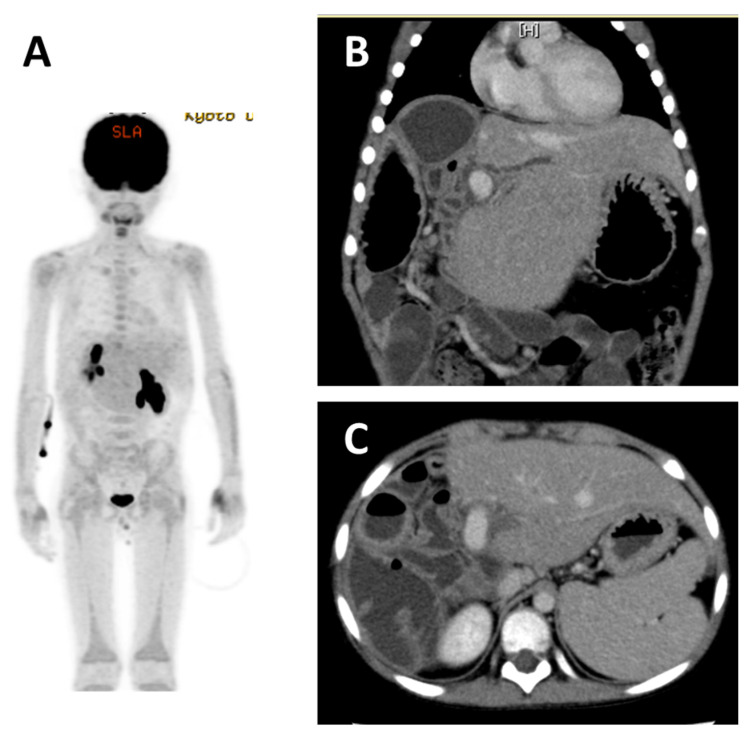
Monomorphic PTLD; DLBCL, post-chemotherapy. Case 2 (continued). Among the immunosuppressive drugs, only tacrolimus was discontinued, and he was treated with rituximab plus B-NHL03. Complete remission was achieved 8 months after the start of treatment. (A) PET-CT confirmed no accumulation of radionuclides. (B,C) CT examination revealed improvement of thickened enlargement of the Roux-en Y jejunum.
